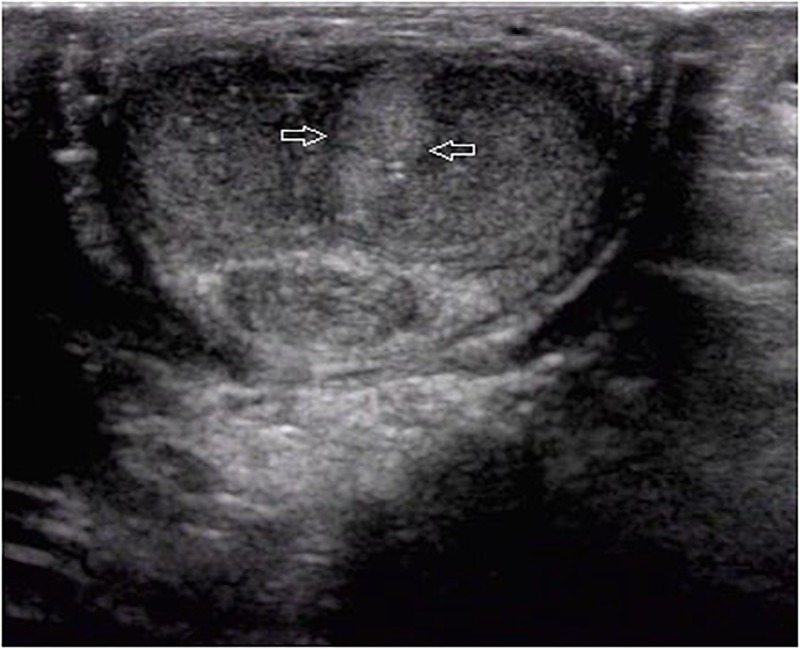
A septal fibrosis in the flaccid penis. Axial sonogram, ventral access, the hyperechoic fibrosis (arrows) in the septum.
